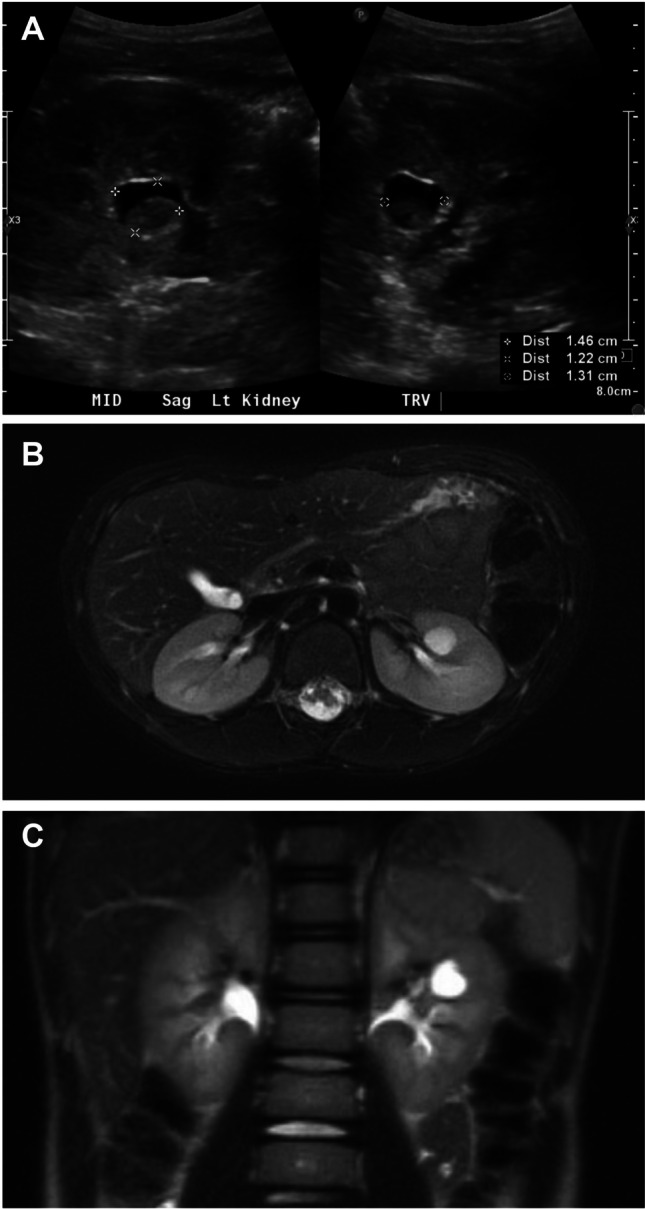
Ultrasound (US) and magnetic resonance urography (MRU) findings of calyceal diverticulum (CD). Imaging studies of a patient with a CD are shown. Ultrasound of the left kidney showing a 1.5 × 1.2 × 1.3 cm anechoic cyst containing layering echogenic debris (A). Axial (B) and coronal (C) T2-weighted MRU images showing a 1.3 × 1.1 × 1.3 cm T2 hyperintense lesion in the anterior interpolar region of the left kidney. The lesion abuts the collecting system and shows homogeneous hyperintensity on excretory-phase post-contrast images, consistent with filling of a CD from the urinary space
